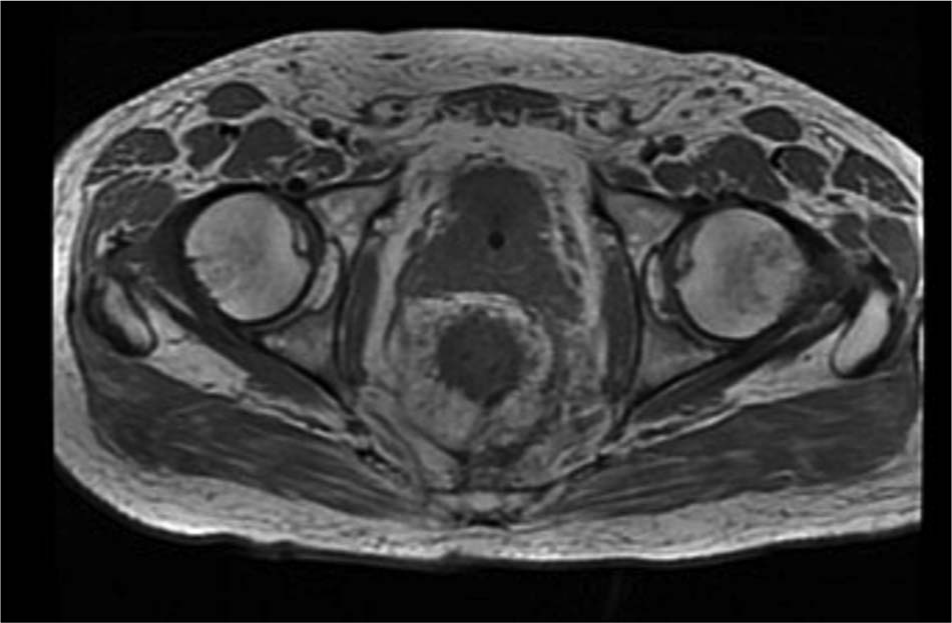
Pelvic MRI staging cT4bN1a.
